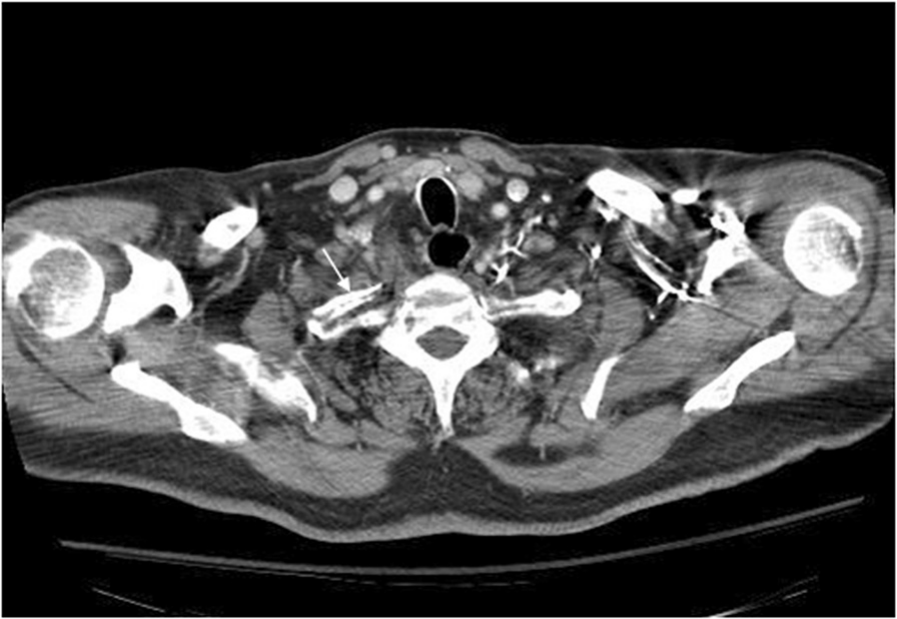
CT of the chest with contrast. This axial view reveals an anteriorly displaced fracture fragment of the proximal right first rib (white arrow).
